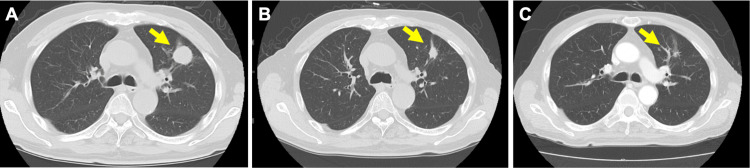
Chest CT images showing the response of the lung tumor to treatment CT: Computed tomography (A) Before treatment: Initial presentation of the lung tumor. (B) After one cycle of combination therapy with carboplatin, paclitaxel, nivolumab, and ipilimumab. (C) After two cycles of maintenance therapy with nivolumab and ipilimumab. Arrows indicate the location of the tumor
